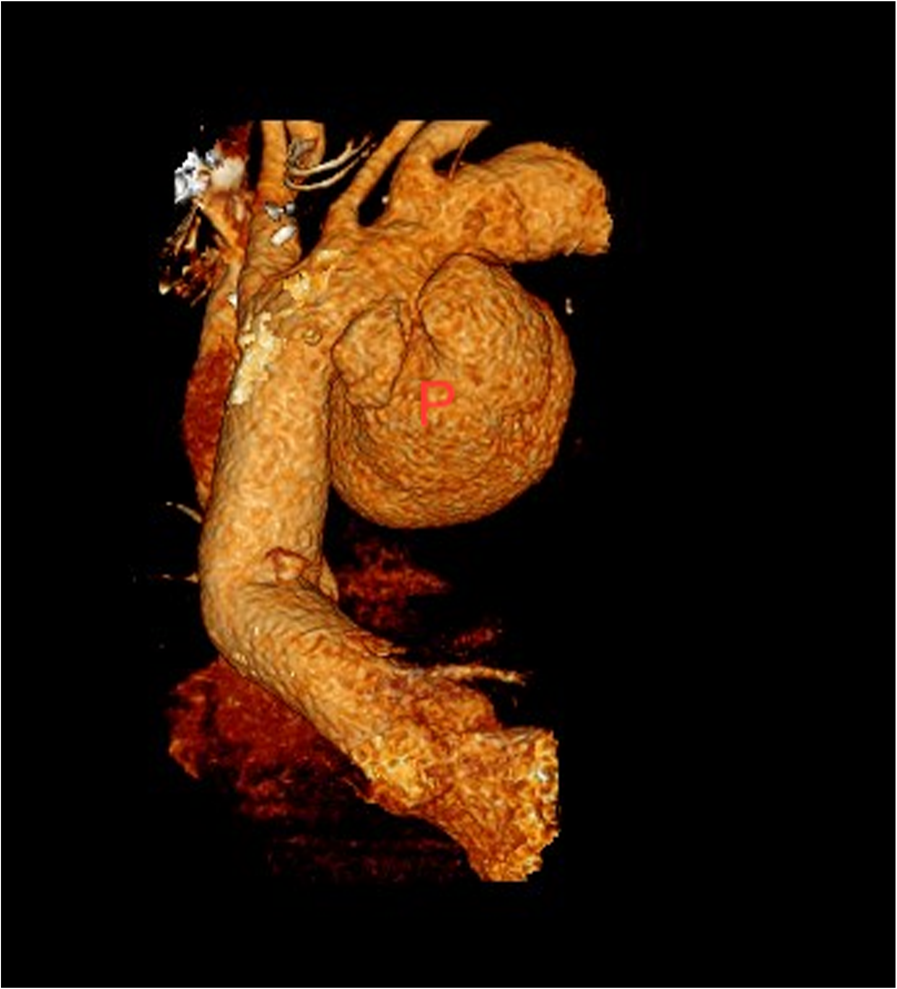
CT scan showing 14 cm pseudoaneurysm arising from the arch of aorta (P)
